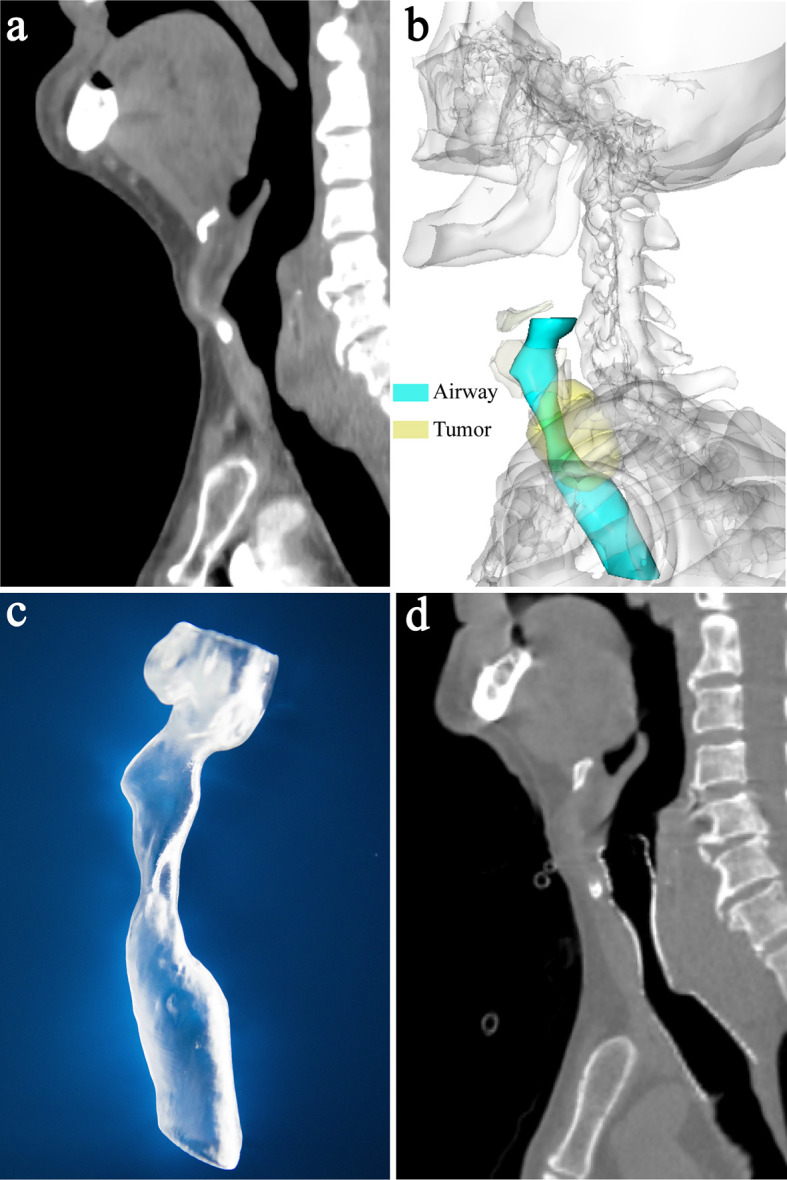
A 87-year-old man with laryngotracheal stenosis caused by thyroid cancer. (A) Sagittal CT showed subglottic and upper trachea stenosis caused by tumor compression before implantation of laryngotracheal stent. (B) 3-dimensional reconstruction image based on CT showed the stenotic subglottic area and upper trachea, and the relationship between them and surrounding structures. (C) The patient-specific model of larynx and upper trachea was made by 3-dimensional printer based on CT data. The diameter of the larynx and upper trachea, the extent and degree of stenosis, and the distance between the proximal end of the stenosis and vocal cord can be shown clearly on the model. (D) Sagittal CT showed that airway patency was maintained 1 month after stenting and the proximal end of the stent was located slightly above the vocal cord and below the epiglottis.
